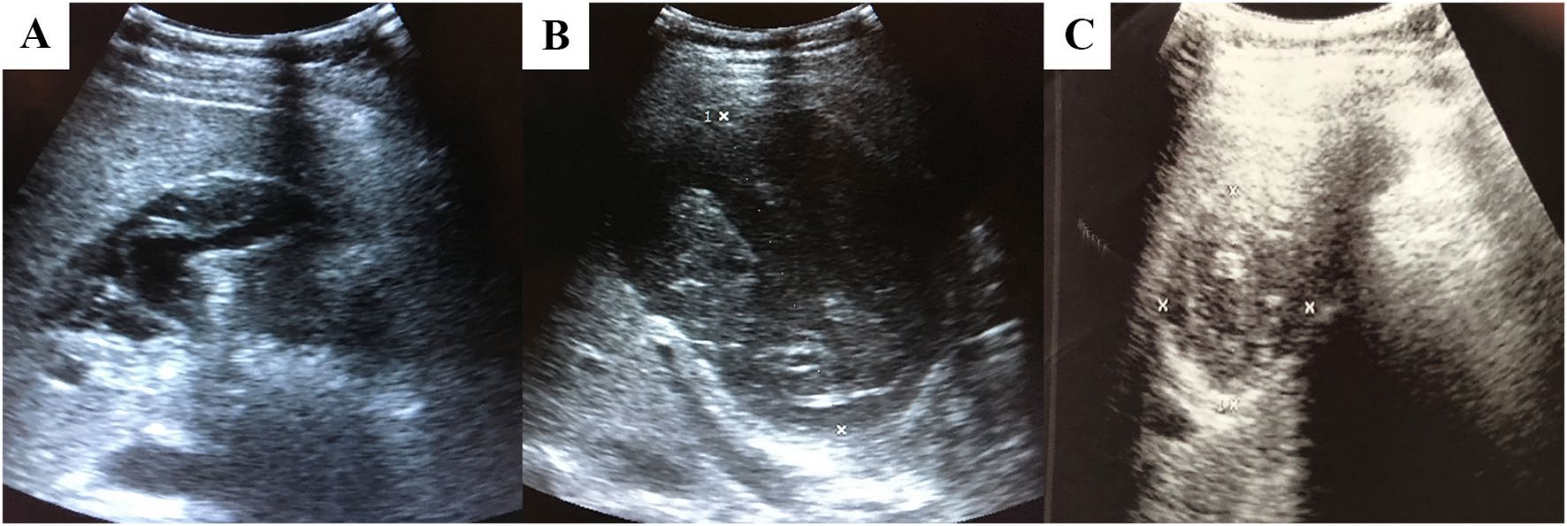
a–c Abdominal ultrasonography: subsequent evolution of the liver cyst after aspiration and combined albendazole—praziquantel therapy. a after having 13 withdrawn 1500 ml of cyst fluid; b one week after removal of the catheter; c twenty-three months after PD
